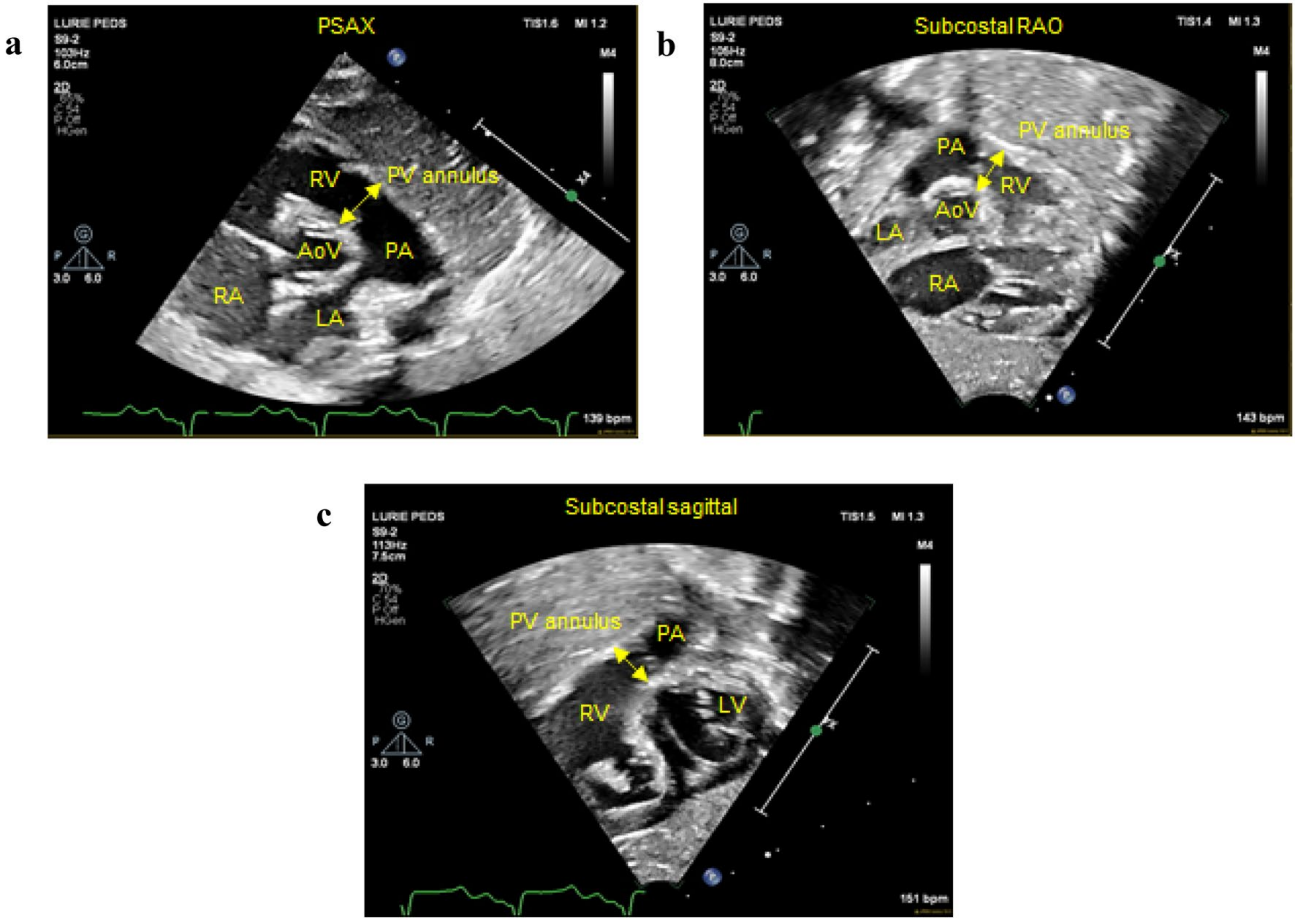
Key echocardiographic views demonstrating measurement of pulmonary valve annulus: a PSAX view, b subcostal RAO view, and c subcostal sagittal view. *PSAX* parasternal short axis, *RAO* right anterior oblique, *RA* right atrium, *TV* tricuspid valve, *RV* right ventricle, *RVOT* right ventricular outflow tract, *PV* pulmonary valve, *PA* pulmonary artery, *LA* left atrium, *LV* left ventricle, *AoV* aortic valve
